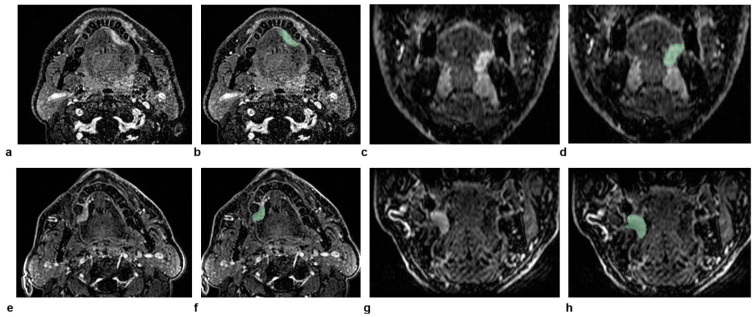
The figure shows dynamic high-spatial-resolution T1-weighted images after contrast medium administration in the axial and coronal planes of two patients with the same radiological staging (cT2N0) but different clinical outcomes, for whom the radiomic-only model and all combined models provided the correct predictions. In the top row (a–d), the images represent a 58-year-old male patient who did not experience a recurrence, with the corresponding delineated lesion outlined in green on the same planes (b,d). In the bottom row (e–h), the images represent a 71-year-old male patient with a recurrence. Similarly, the axial and coronal images (e,g) show the lesion after contrast medium administration, with the delineated lesion outlined in green (f,h).
